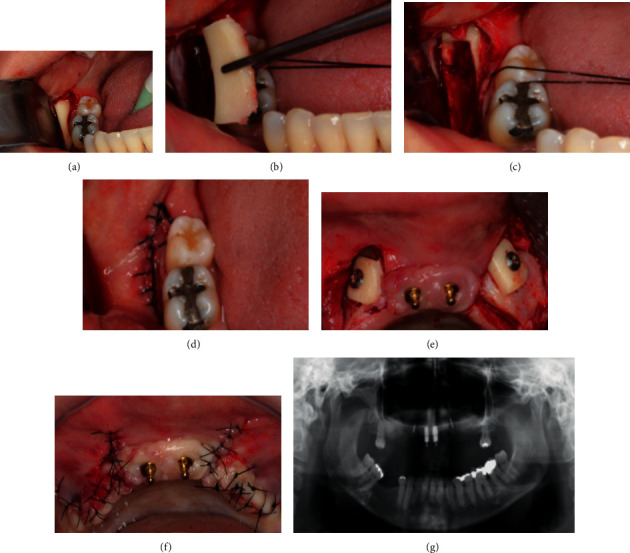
The third surgical approach was to install an onlay autogenous block bone graft harvested from the mandibular ramus. The graft was split and was installed in the receptor area in the posterior maxilla to augment the alveolar crest width and consequently to allow implant installation in an ideal tridimensional position (a–f). Panoramic radiography was taken immediately after graft placement (g).
